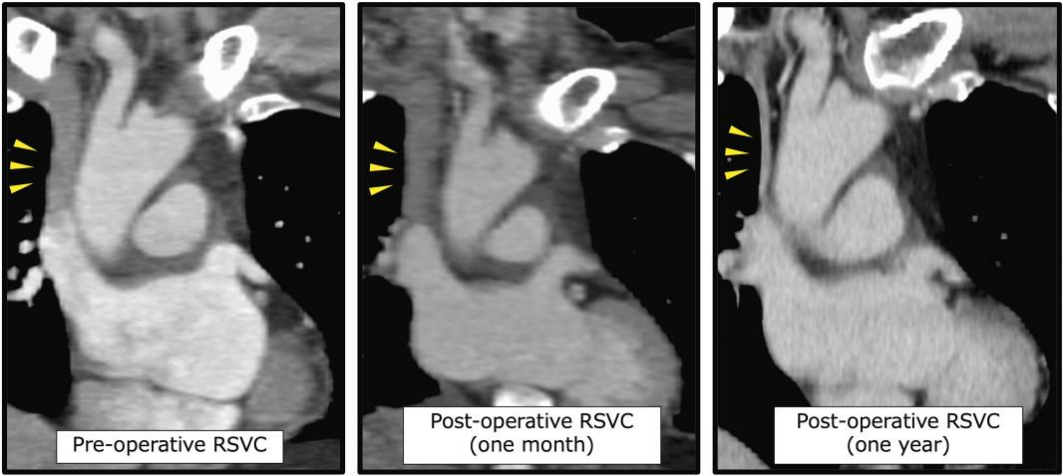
Follow-up contrast-enhanced CT images of the RSVC (yellow arrows) before and one month and one year after ligation.
